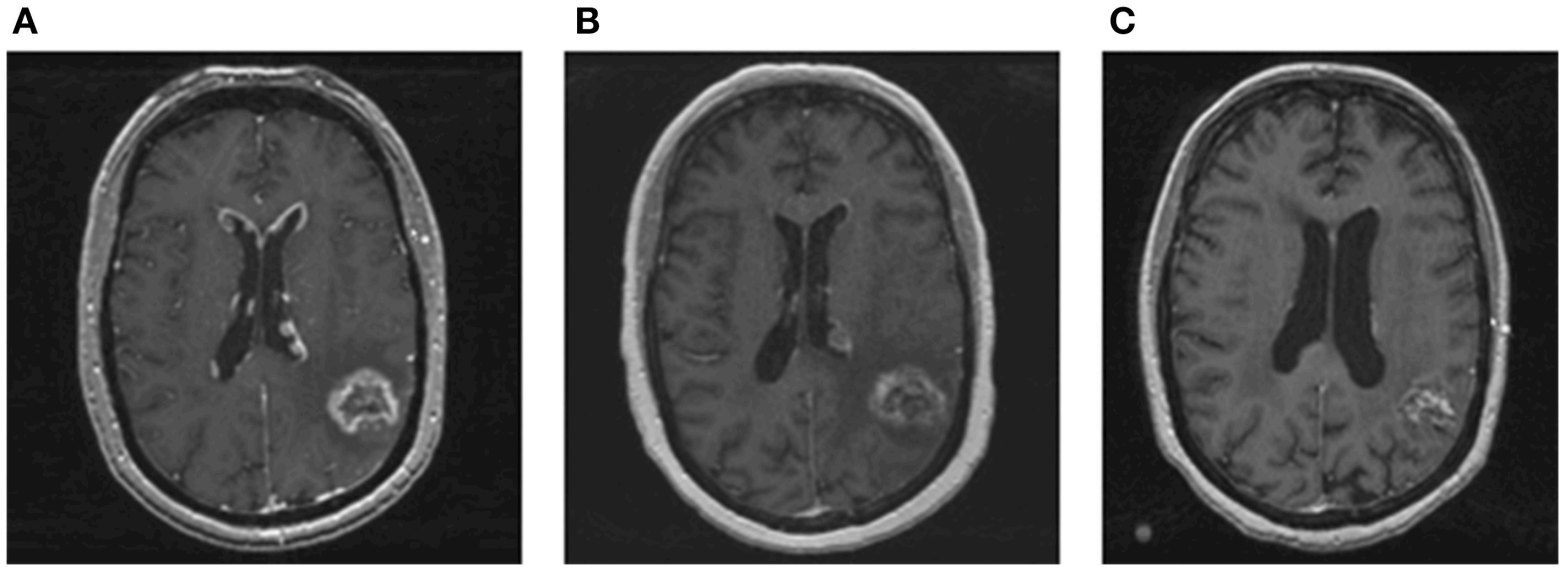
MRI images of ependymal disease and response to whole brain re-irradiation. (A) Contrast enhanced T1 MRI showing new enhancing, linear, and nodular lesions along the ventricles consistent with ependymal disease. (B) Contrast enhanced T1 MRI performed 2 months after whole brain re-irradiation showing a significant and rapid decrease in ependymal enhancement. The treated metastasis in the left temporoparietal area was stable. (C) Contrast enhanced T1 MRI performed 1 year after whole brain radiation showing resolution of nodular lesions along the ventricles and no new brain metastases.
